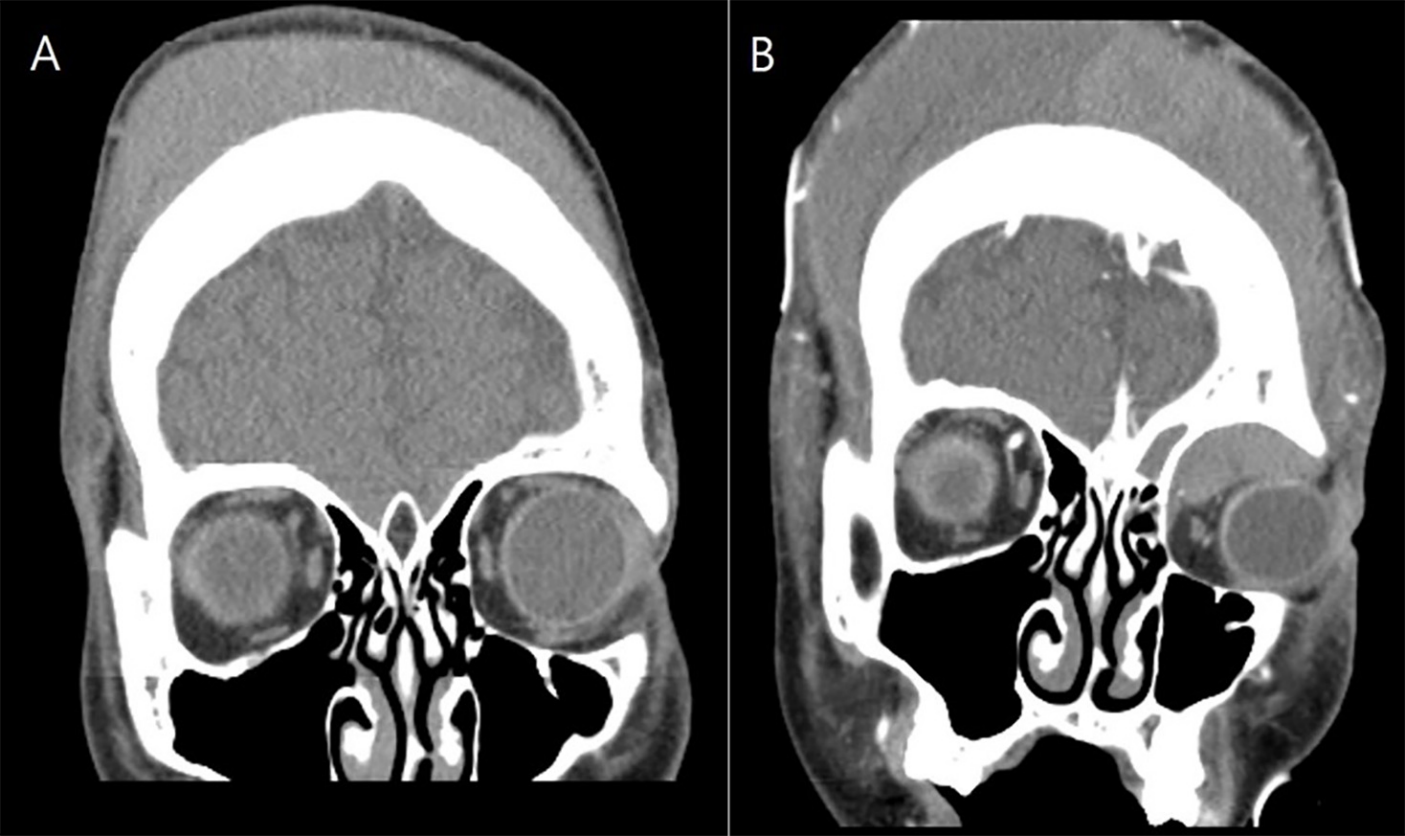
Orbital CT scans. (A) CT performed four days before hspitalization. A subgaleal hematoma and swelling near the left orbit were observed, but no intraorbital hematoma was observed. (B) CT taken at the time of hospitalization. Subgaleal hematoma was observed in the right frontal, occipital, and left temporal regions. A suspected hematoma was observed in the left intraconal space, and left eye proptosis and deviation became worse compared to 4 days ago.
